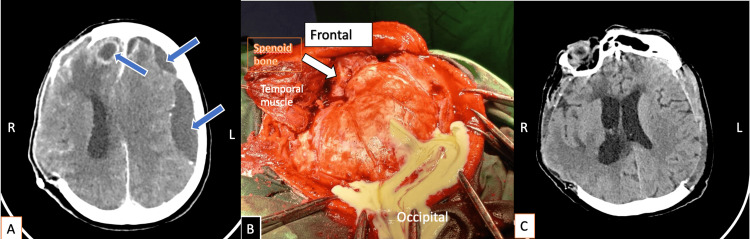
(A) Contrast-enhanced postoperative CT performed two to three days after the first surgery demonstrates enlarging subdural collections over the left hemisphere, predominantly along the convexity, with the largest collection in the fronto-temporo-parietal region and several smaller frontal collections. An associated left frontal intracerebral abscess with features of evolving cerebritis is indicated (blue arrow). (B) Intraoperative findings show purulent discharge following minimal dural incision during wide decompressive craniectomy. (C) Postoperative imaging demonstrates the result of bilateral wide decompressive craniectomy, with persistent cerebral edema but near-complete resolution of the mass effect and midline shift. CT = computed tomography; L = left; R = right
